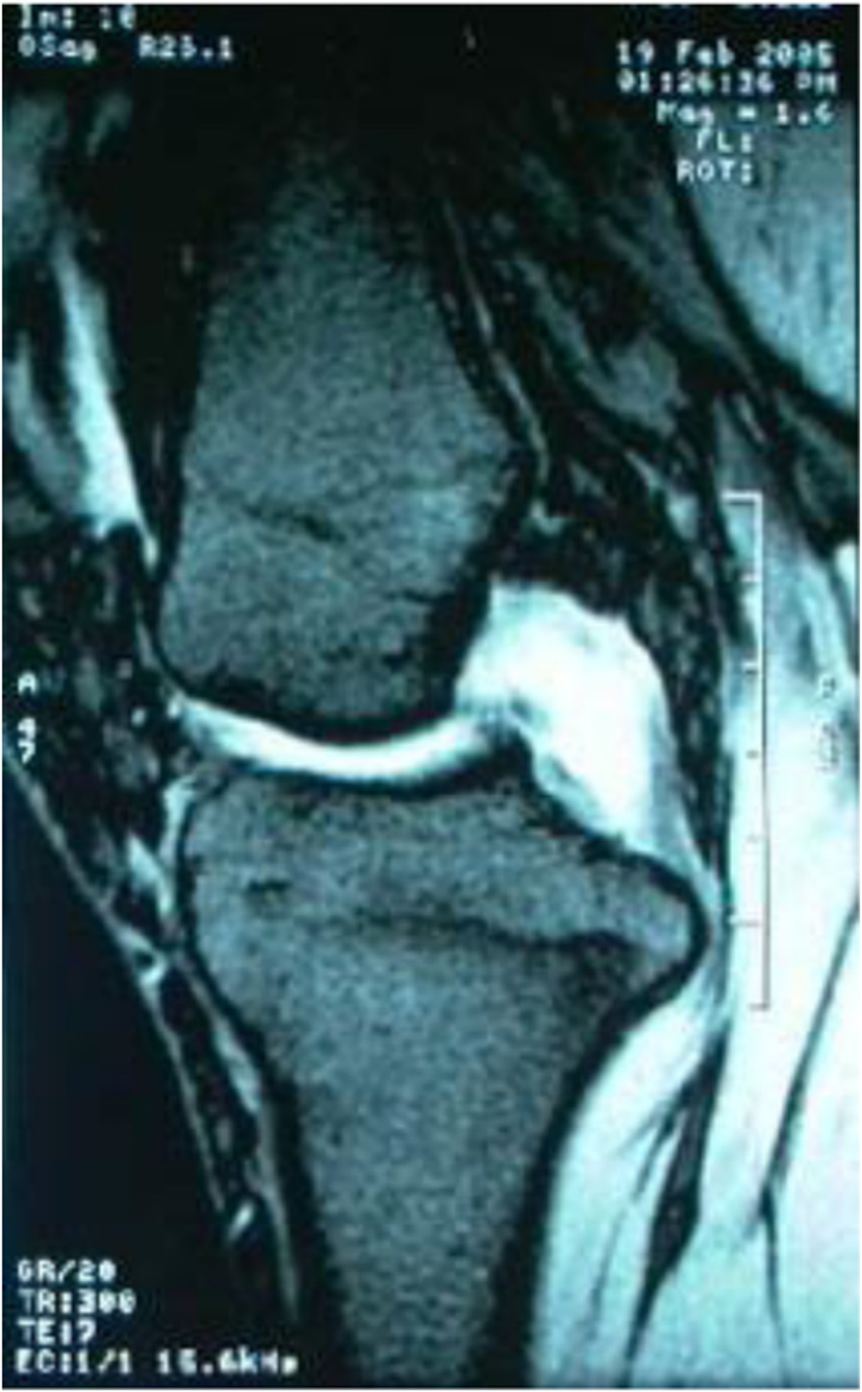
Contrast MR image showed slightly heterogeneous moderate enhancement and hypo-intensity ringlike non-enhancement.
